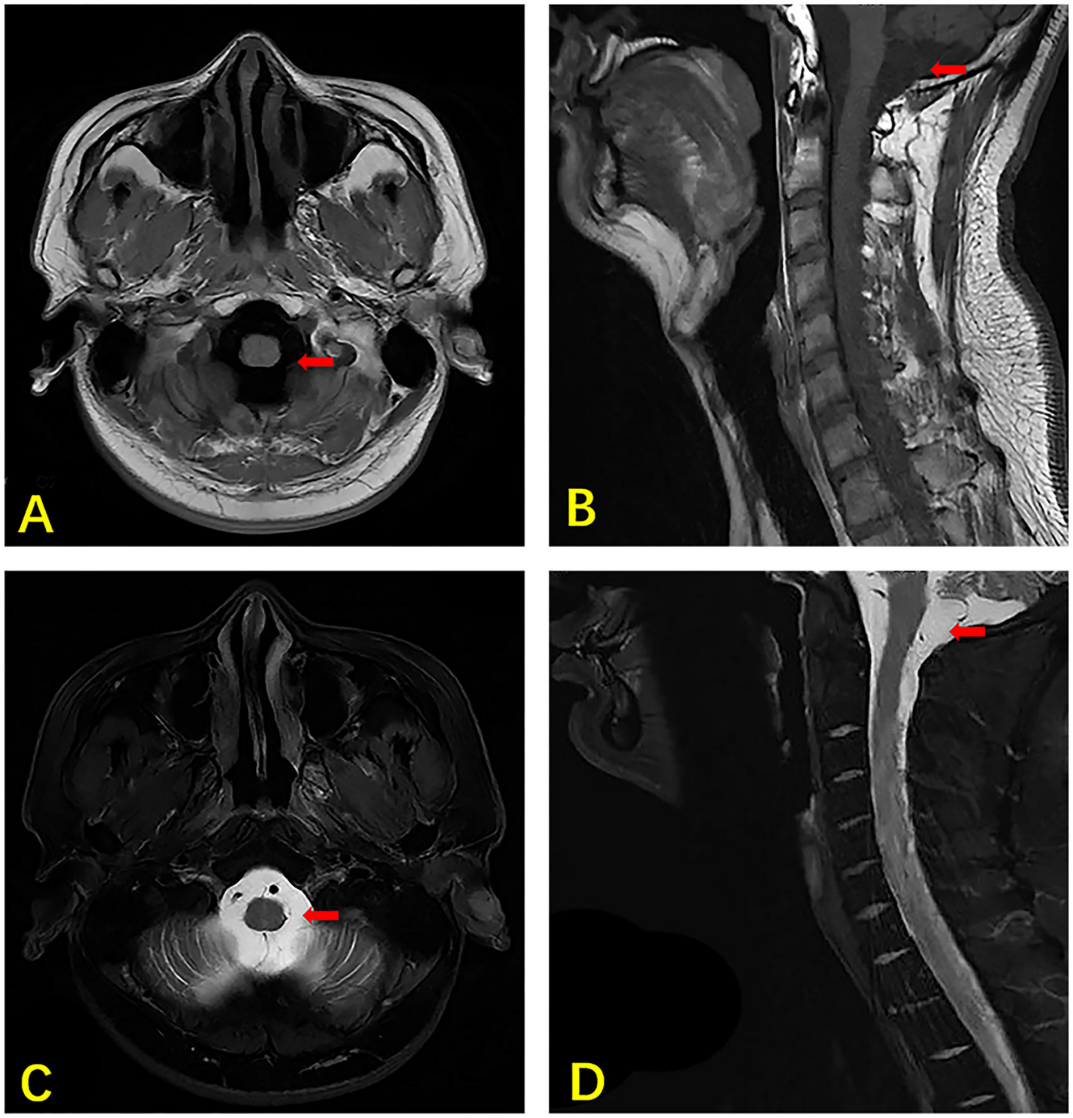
MRI of the brain and cervical vertebra of our case. The axial section T1 weighted image showing hydrocephalus (arrow) (A). The sagittal section T1 weighted image showing hydrocephalus (arrow) (B). The axial section T2 weighted image shows hydrocephalus (arrow) (C). The sagittal section T2 weighted image showing hydrocephalus (arrow) (D).
